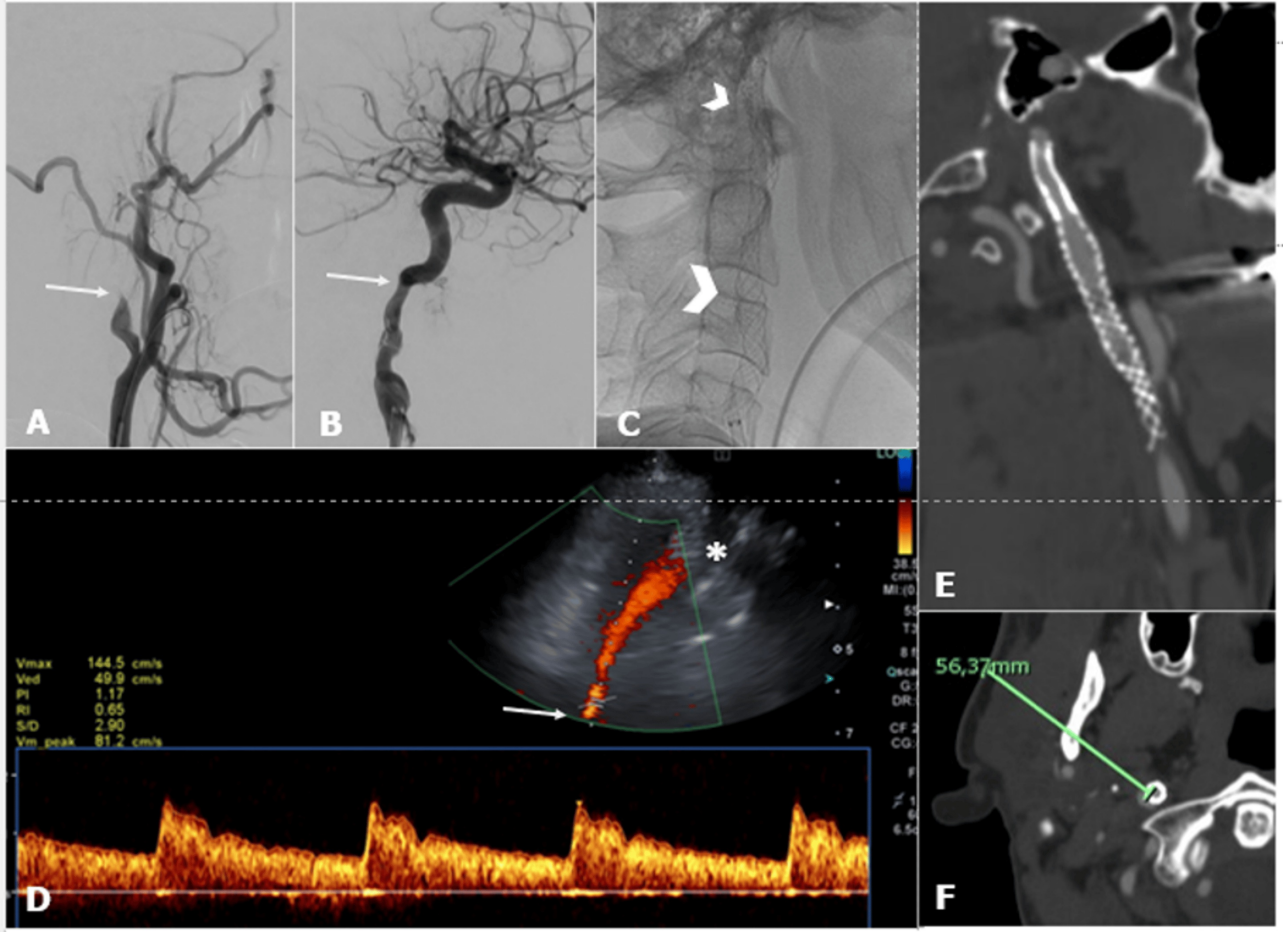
High cervical ICA stenting in tandem stroke. Right cervical internal carotid artery (ICA) dissection before (A) and after (B) mechanical thrombectomy (arrows). Acute double stenting from the carotid bifurcation to the pre-petrous ICA segment (arrowheads) (C). Color Doppler US with transcranial probe at three-month stenting follow-up (D): 6-cm depth pre-petrous ICA segment color Doppler sampling (white arrow) with velocities within limits; also, stent meshes are visible (asterisk). Six-month CT angiography (E, F) confirming stents' integrity and patency; see ICA depth in CT (F).
